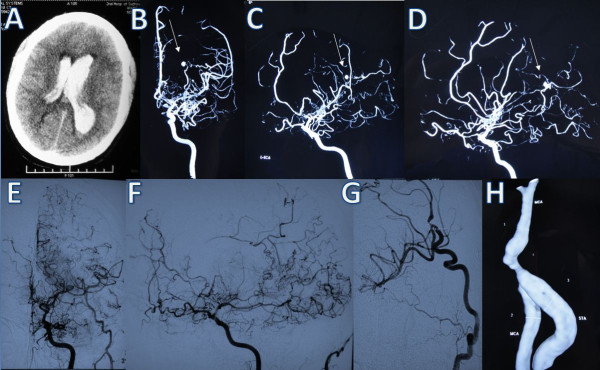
Case 2. (A) Computed tomography scan showing diffuse intraventricular hemorrhage. (B), (C), (D) Right carotid artery angiograms obtained after the hemorrhage showing marked stenosis of the proximal segment of the middle cerebral artery with a diffuse network of collateral fine vessels. There is an aneurysm (arrows) arising from the left posterior choroidal artery (B frontal view, C oblique view, D lateral view). (E), (F), (G), (H) DSA image at postoperative follow-up showing no residual aneurysm and an enlarged STA.
